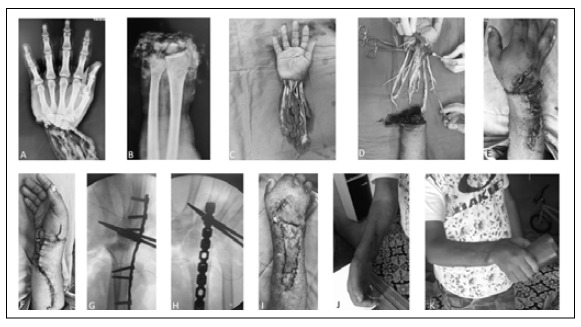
Case 4: (A and B) X-rays of the wrist and amputated hand; (C) Appearance of the hand amputated by avulsion; (D) Debridement of non-viable tissue; (E and F) Final appearance after surgery; (G and H) Radioscopy imaging after wrist arthrodesis; (I) Image after skin graft surgery showing good integration; (J) Clinical image of the upper limb at follow-up; and (K) Patient holding an object.
